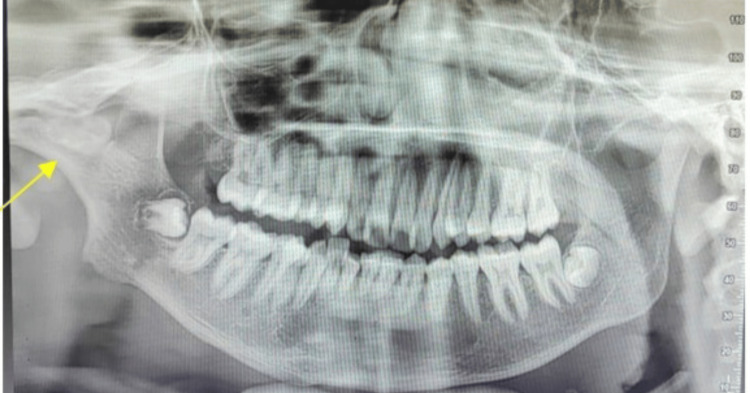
Preoperative orthopantomogram showing right-sided TMJ ankylosis.
